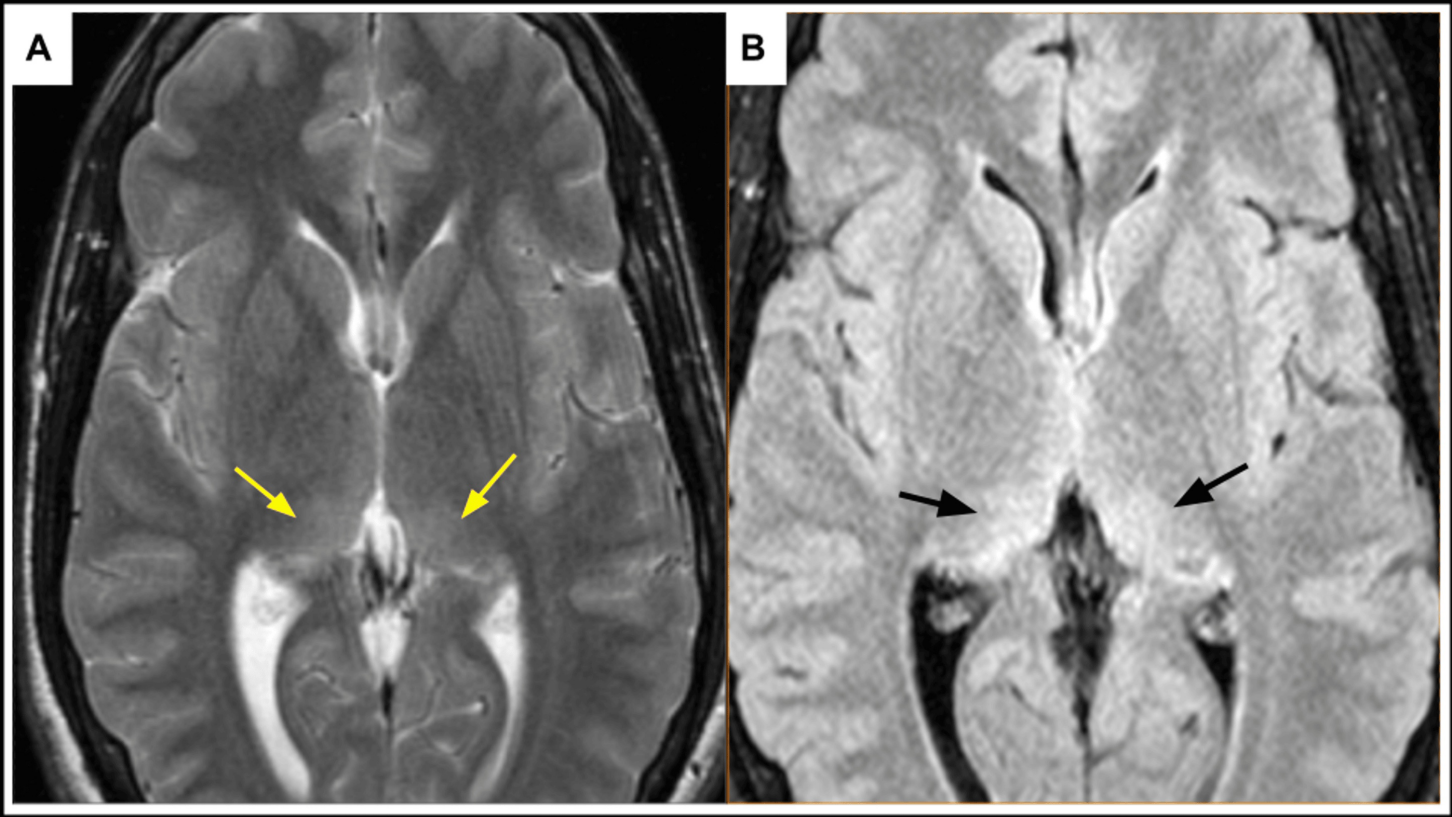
Magnetic resonance imaging of the brain demonstrates FASI within the medial thalami bilaterally on T2-weighted (yellow arrow) (A) and fluid attenuation inversion recovery (black arrow) (B) sequences. FASI: focal areas of signal intensity
